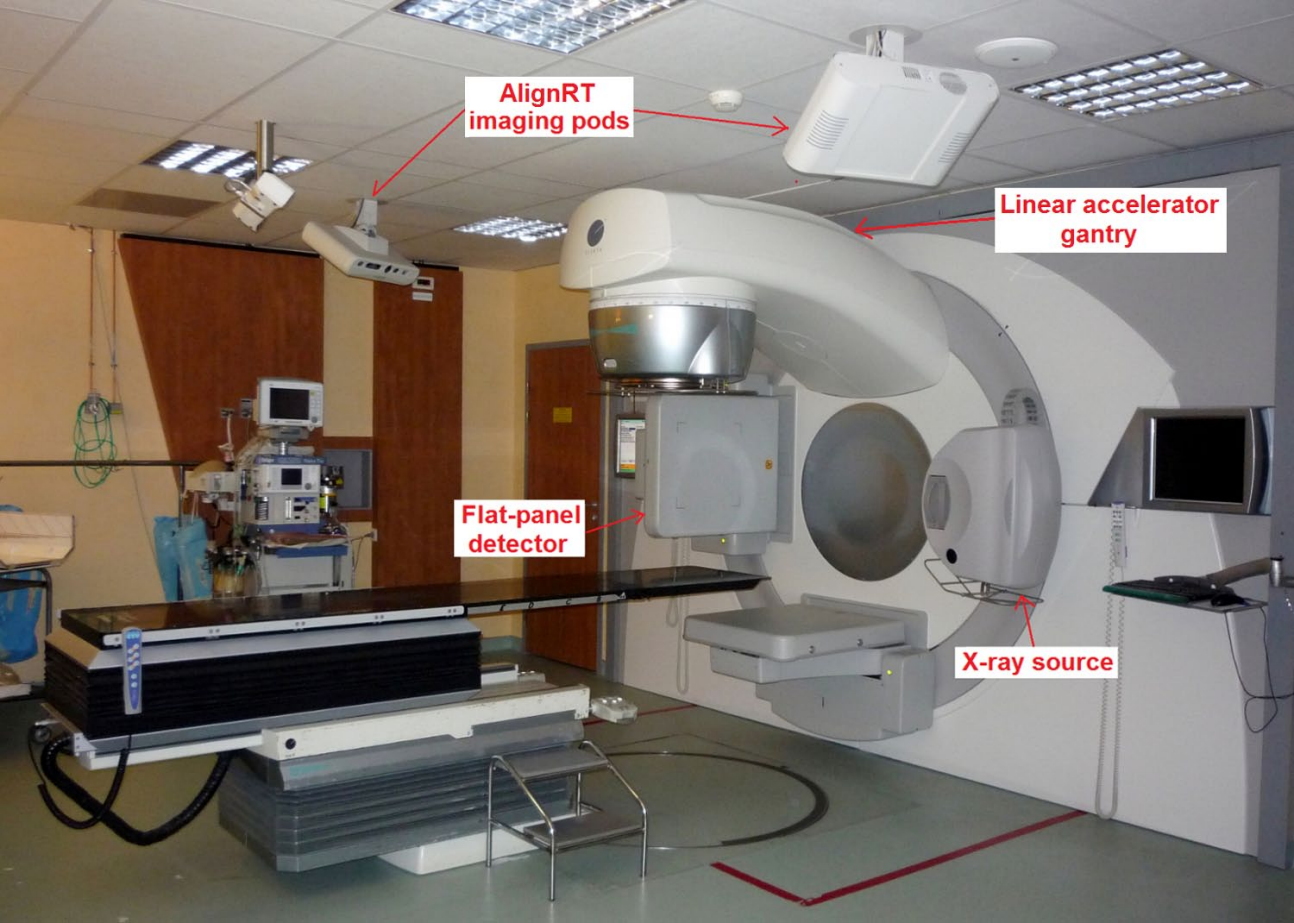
Treatment room at CLB where the Elekta Synergy and AlignRT systems are installed. The figure depicts the AlignRT camera pods for optical surface tracking and the X‐ray source and flat‐panel detector for CBCT imaging.
